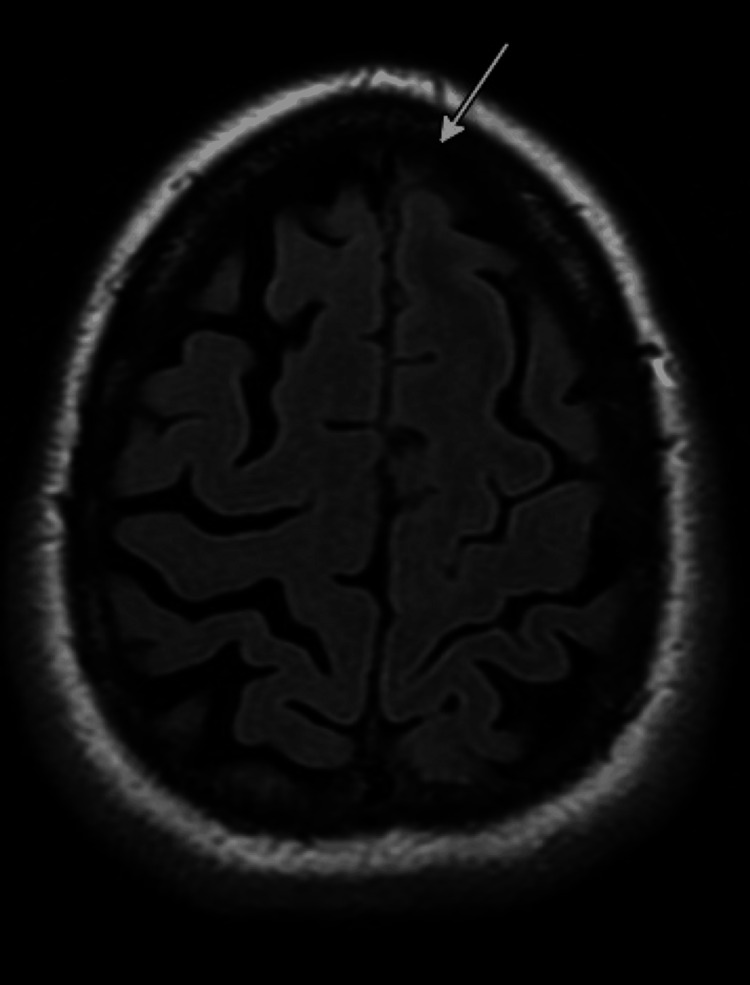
MRI Brain With Arrow Showing Left Meningeal Enhancement MRI: Magnetic Resonance Imaging
